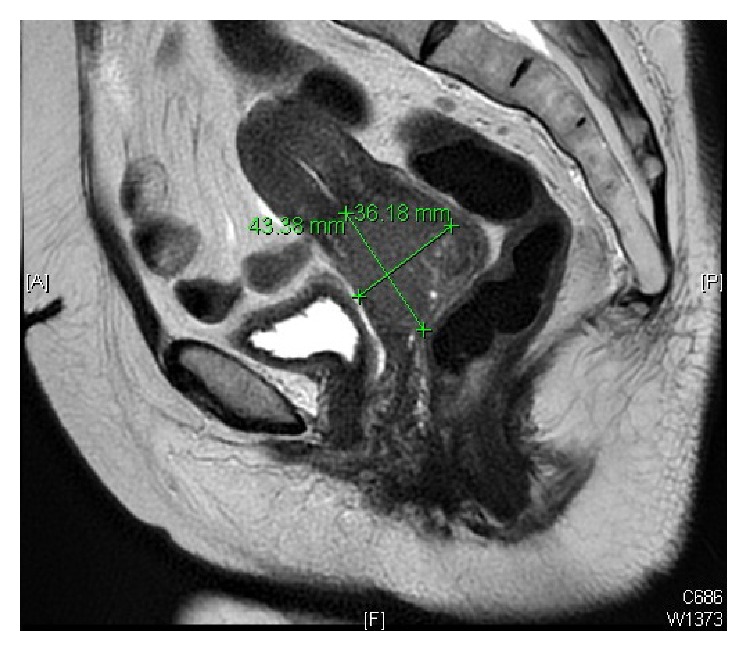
Sagittal view of pelvic MRI showing 4.3 × 3.6 cm cervical mass extending to the lower uterine segment (LUS) and left vaginal fornix with evidence of parametrial invasion on the left side.
